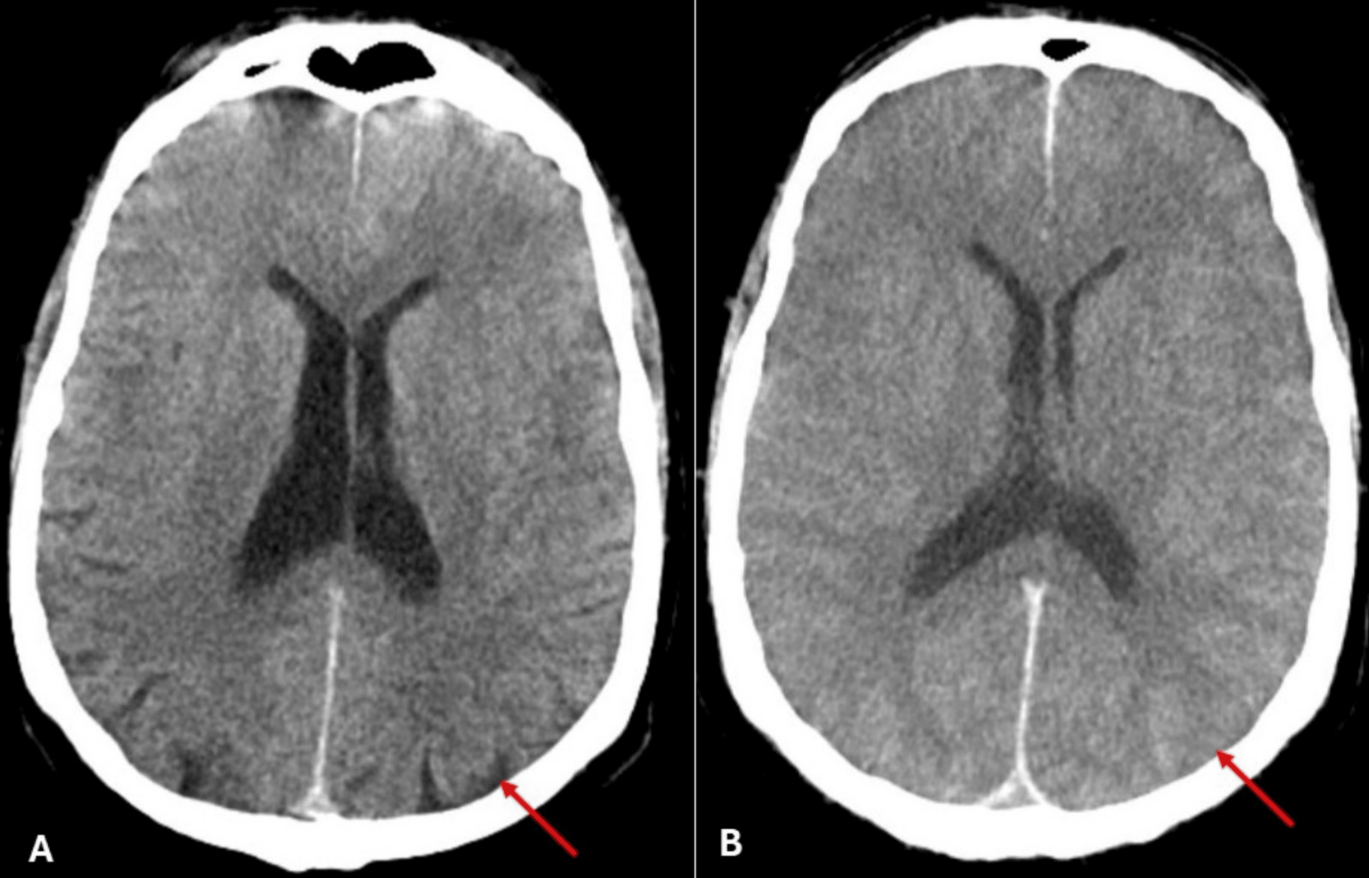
Comparison between non-contrast brain CT at admission (A) and at the 20-hour revaluation (B). (A) Admission CT showing diffuse cerebral oedema, but with preservation of brain sulci and gyri (red arrow); (B) Revaluation CT showing worsening of diffuse cerebral oedema, with effacement of the brain sulci and gyri and with loss of grey and white matter differentiation (red arrow). CT: computed tomography
